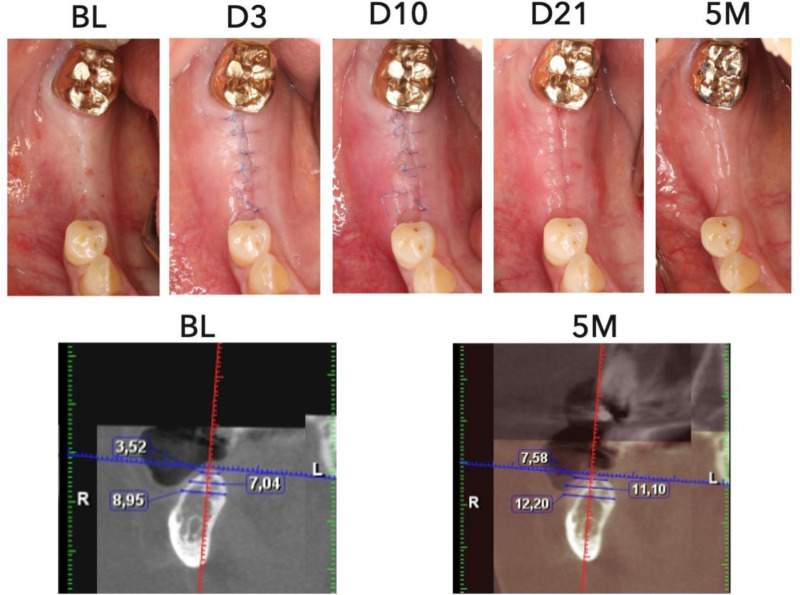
Case demonstration of favorable healing after guided bone regeneration procedure. Top row shows clinical intraoral photography from baseline (BL), 3 days (D3), 10 days (10D), 21 days (D21), and 5 months (5M). Bottom row shows CBCT bone width measurements at baseline (BL), 5 months (5M). There is clear evidence of superior bone gain seen in clinical photos over time due to optimal healing and shown in bone gain measurements in the follow-up CBCT.
